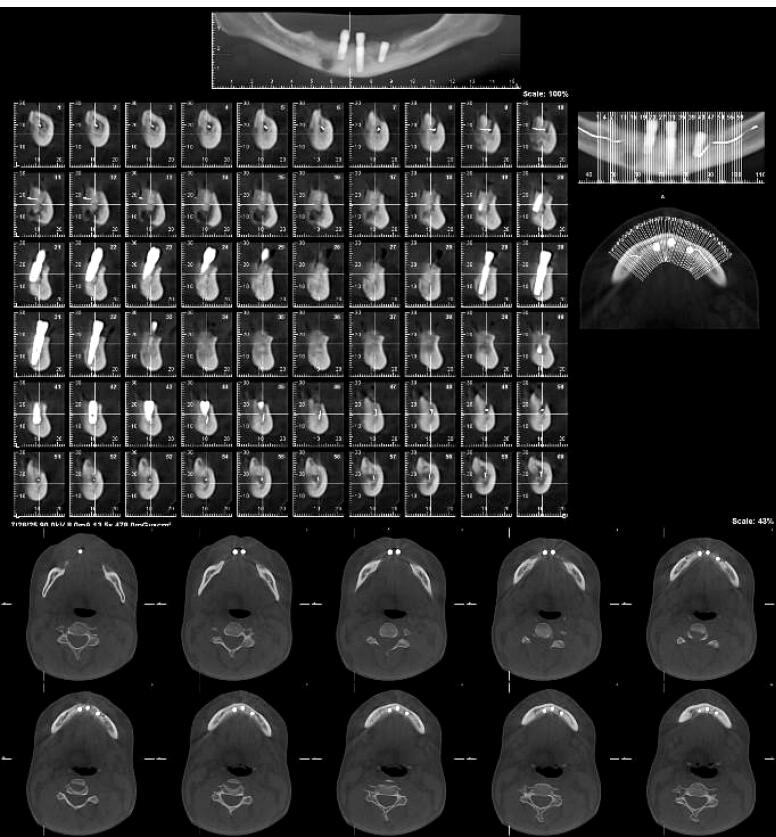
Based on the clinical and radiographic assessment, chronic osteomyelitis secondary to implant infection was considered in the differential diagnosis. Consequently, after comprehensive evaluations, a decision was made to remove the implants. Written informed consent was obtained from the patient for publishing this case report and the accompanying clinical images.
